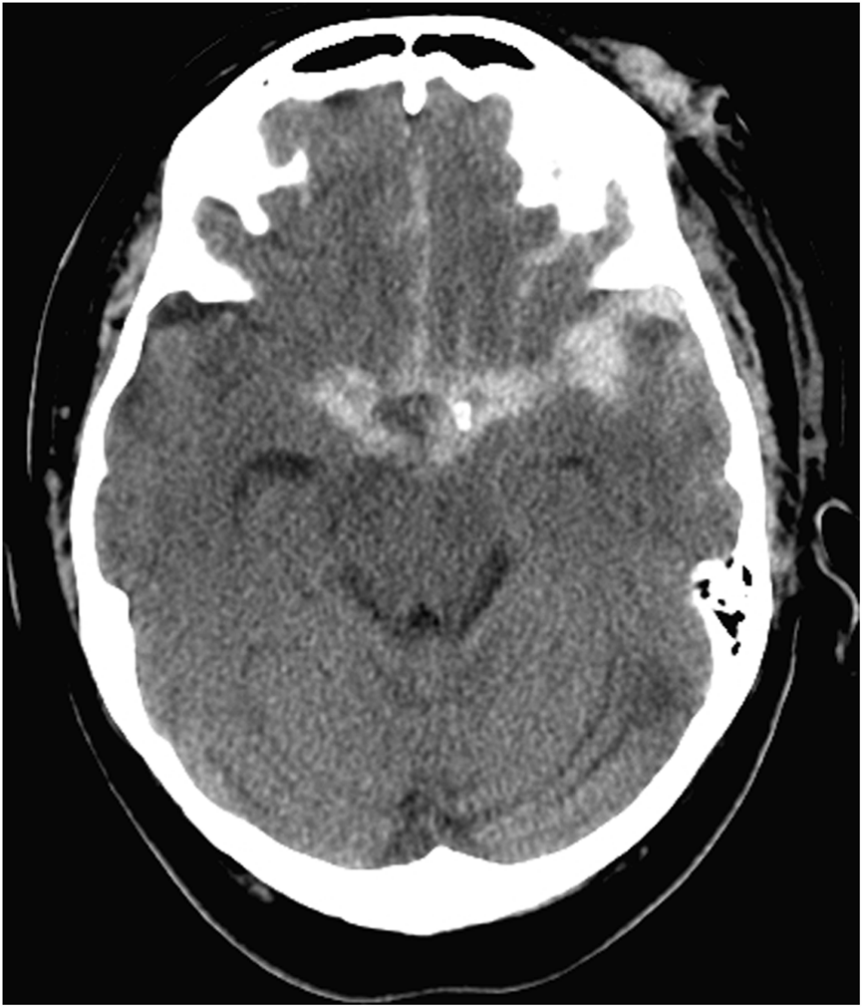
CT-Head demonstrating diffuse subarachnoid hemorrhage in bilateral basal cisterns which is mainly concentrated in the left sylvian fissure (Fisher Grade 3).
